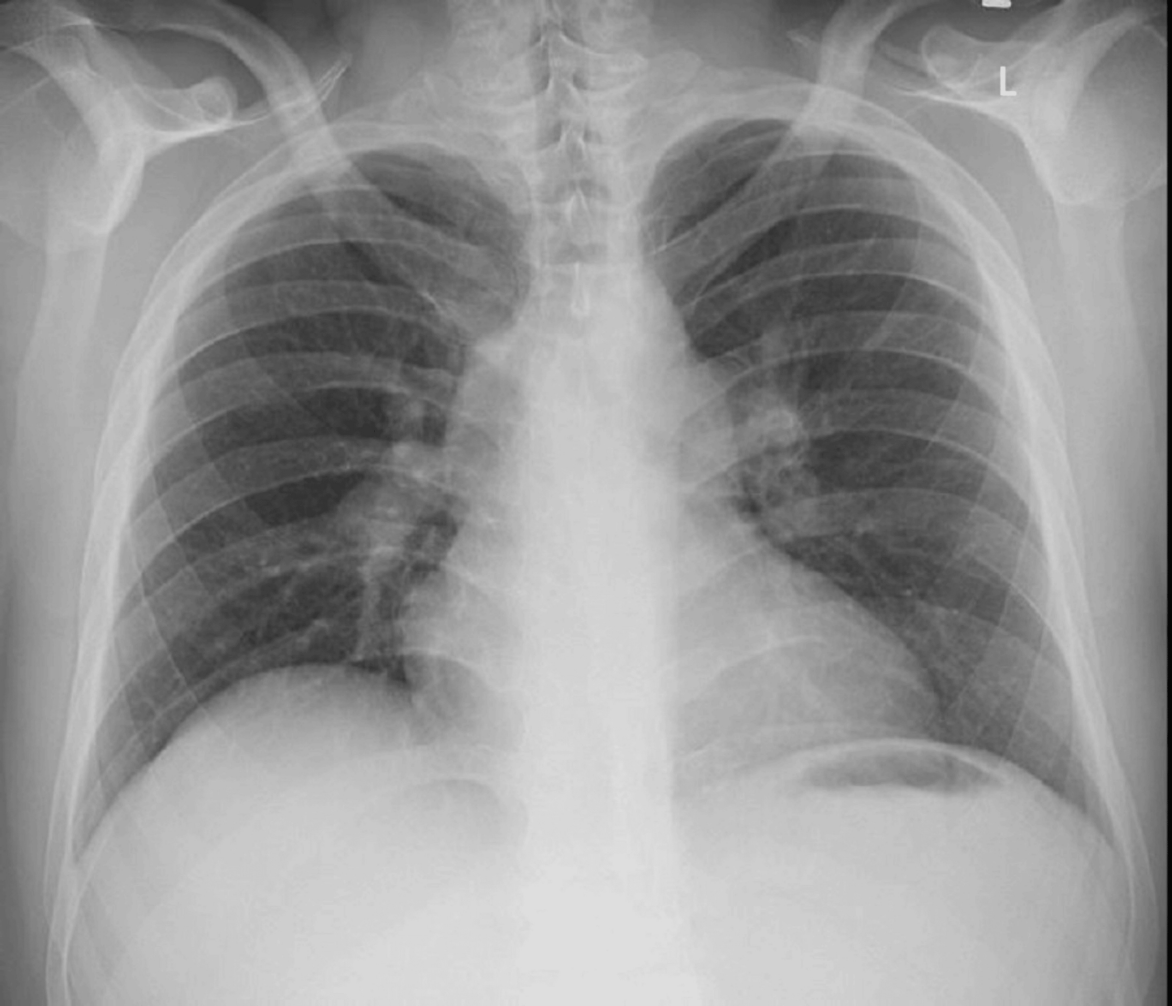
Chest radiograph in posteroanterior view with no significant abnormality
